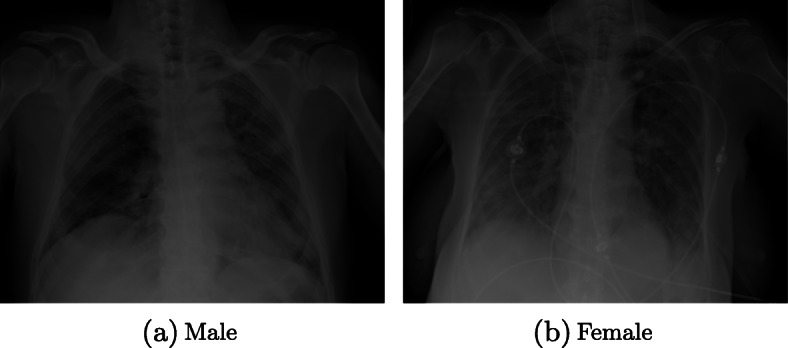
Example of two representative chest X-ray images of male and female patients diagnosed with COVID-19
